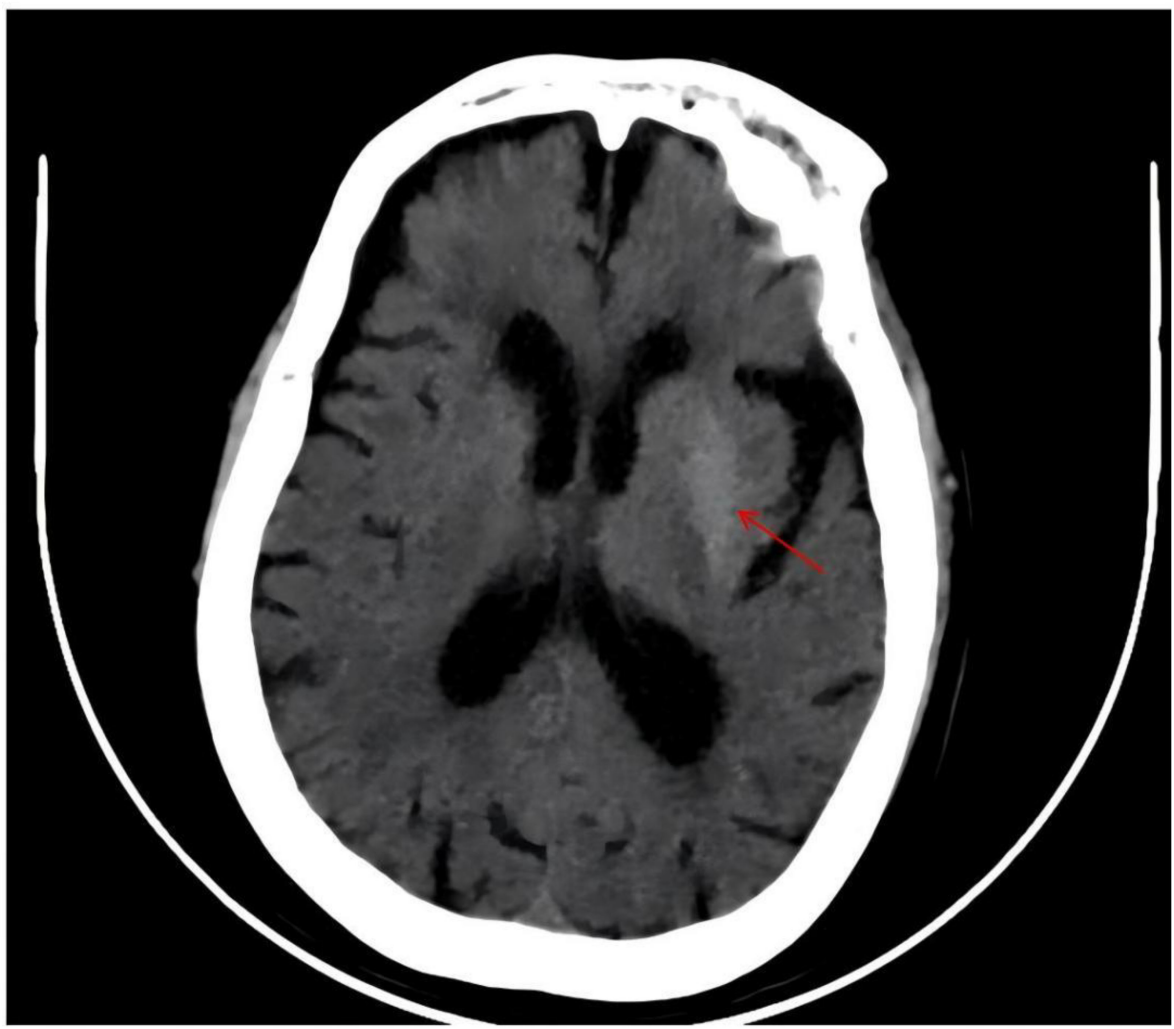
The CT scan reveals increased density in the left basal ganglia, with a lesion measuring approximately 3.5x1.1 cm, and a central CT value of approximately 33 HU. Abnormal signals are marked by red arrows.
